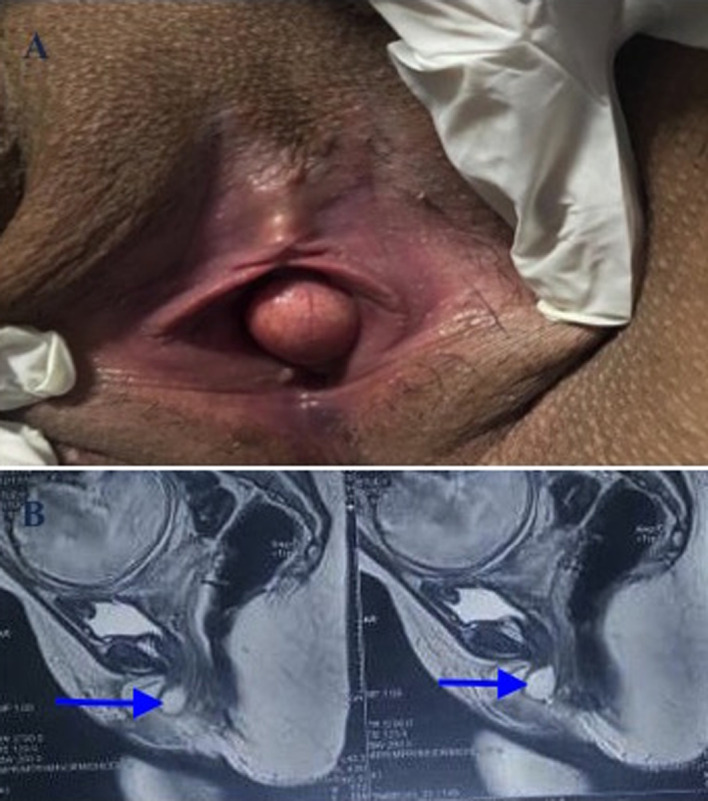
A) clinical appearance of the urethral diverticulum; B) sagittal section of MRI in T2 sequence showing the precise location of the urethral diverticulum
